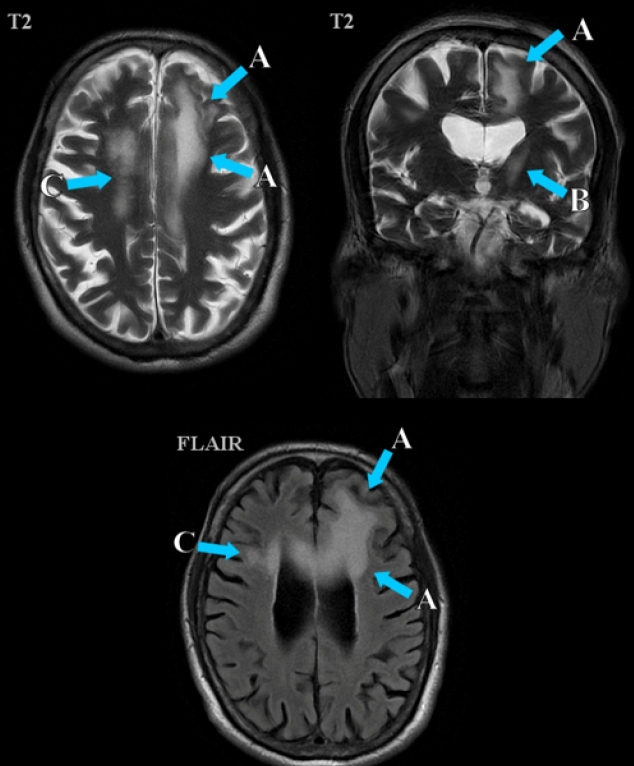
Brain MRI showing an extensive hyperintense lesion on T2 and FLAIR images in the left frontal parasagittal cortico-subcortical (A) and striatocapsular regions (B) and right frontal subcortical area (C).
